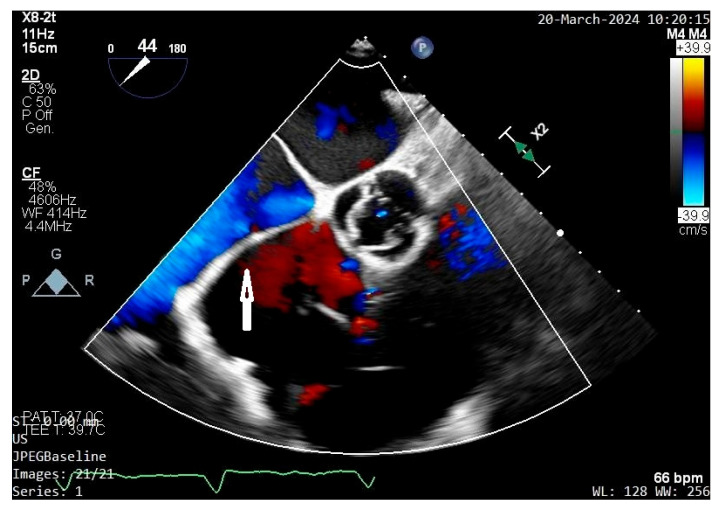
Transoeasophageal echocardiography displaying the cor triatriatum dexter membrane (white arrow) subdividing the right atrium into two chambers.
